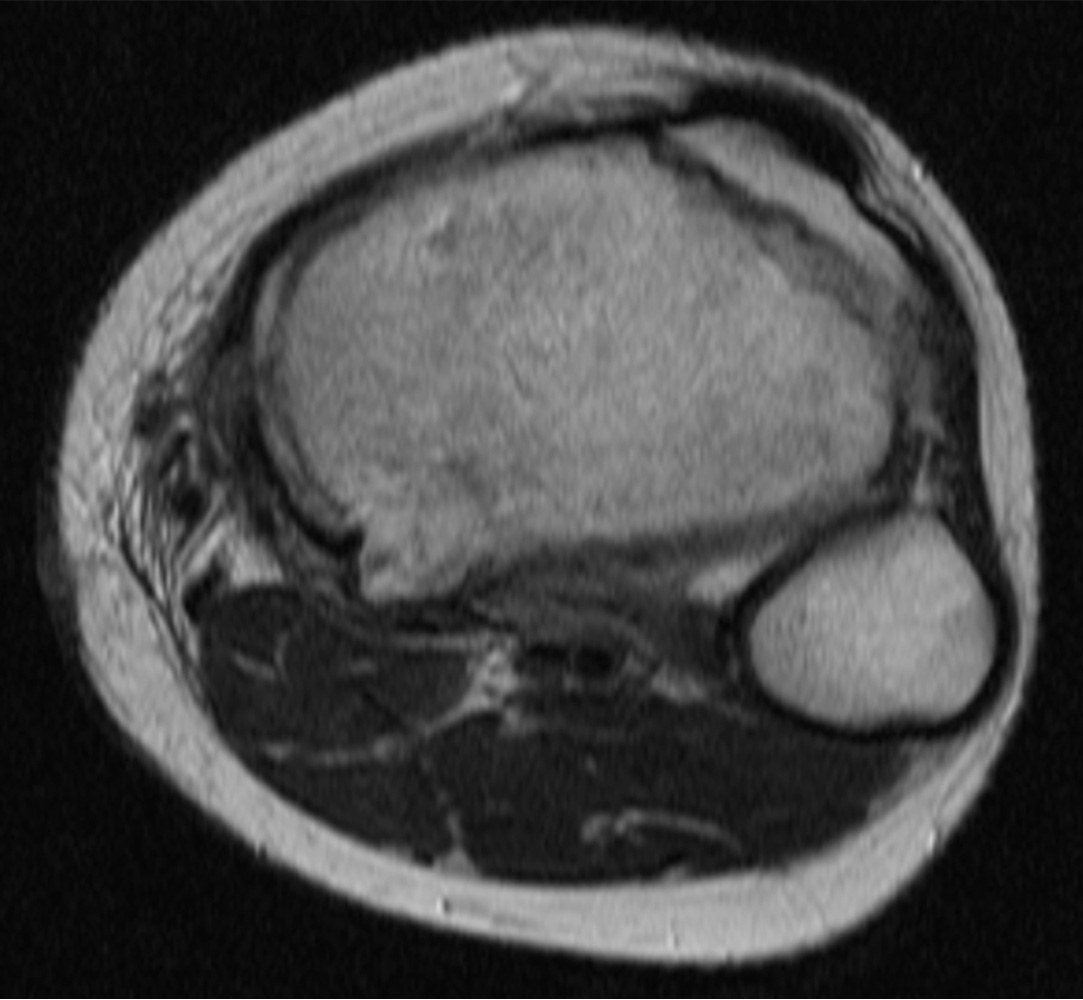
MR imaging at most recent visit. There was no tumor recurrence.
